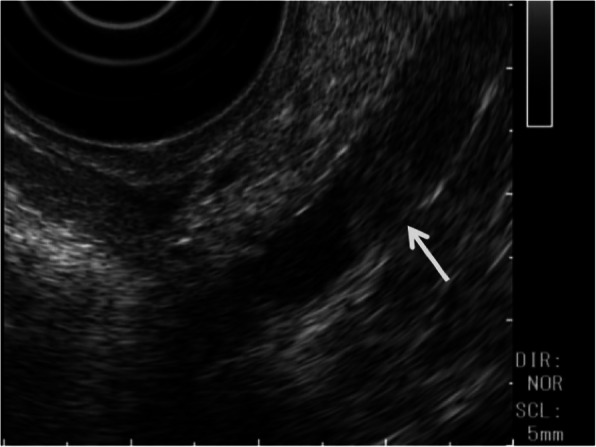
Endoscopic ultrasonography demonstrated an elevated lesion (arrow) of DBD
